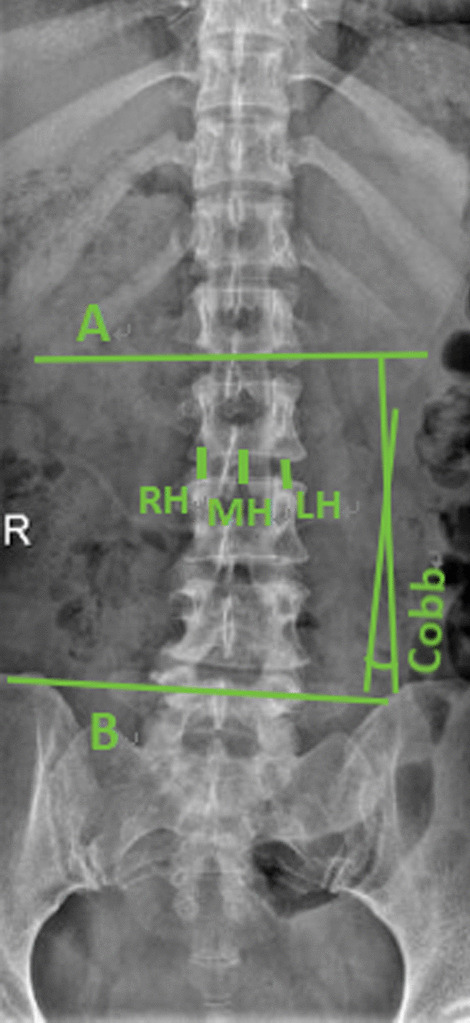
Measurement of coronal Cobb angle and height of intervertebral space on coronal X-ray of lumbar spine. The coronal Cobb angle (C-Cobb) refers to the angle between two straight lines perpendicular to A and B, A is the parallel line to the most inclined vertebral endplate at the head end, and B is the parallel line to the caudal most inclined vertebral endplate. The height of intervertebral space in coronal position (C-ISH) = (RH + MH + LH)/3, RH, MH and LH are the heights of right, middle and left intervertebral space, respectively
